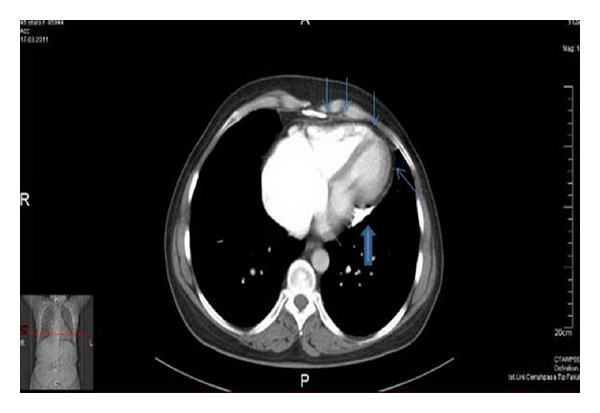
Pericardial thickening (thin arrows) and heavy calcification of left atrioventricular groove (thick arrow) in cardiac CT.
